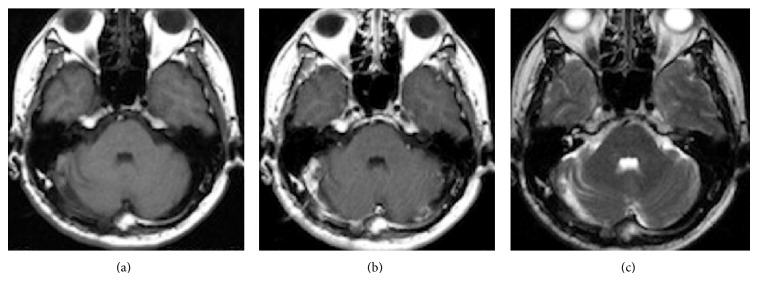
Postoperative T1-weighted plain (a), gadolinium-enhanced (b), and T2-weighted (c) MRI with contrast medium suggesting complete removal of the tumor without recurrence at 9 months postoperatively.
